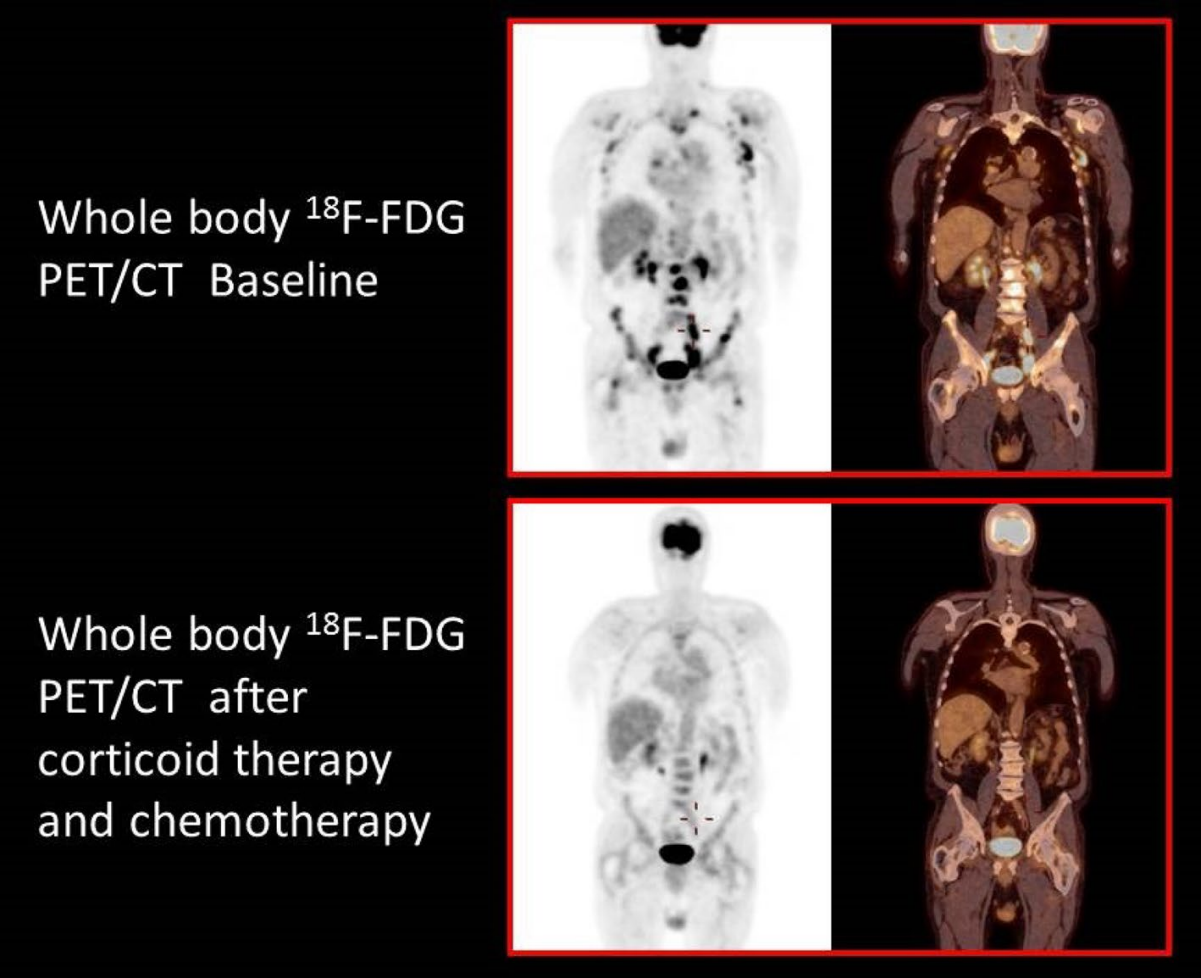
Whole body ^18^F-FDG PET/CT demonstrating a complete response after corticoid therapy, 6 cycles of R-CHOP, and 4 cycles of HD-MTX
